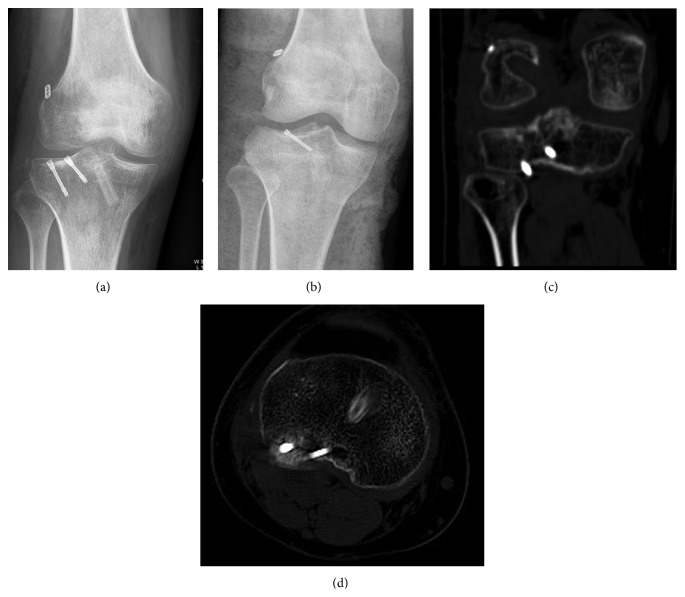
X-ray images of the knees after the operation. In the first patient (a), button steel was used at the femoral site for ACL reconstruction and two lag screws were used for posterolateral tibial fracture fixation. In the second patient (b), similar procedure to that for the first patient, but only one screw, was used. (c and d) The CT scans in patient one showed the tibial plateau fracture was well reduced and fixed.
